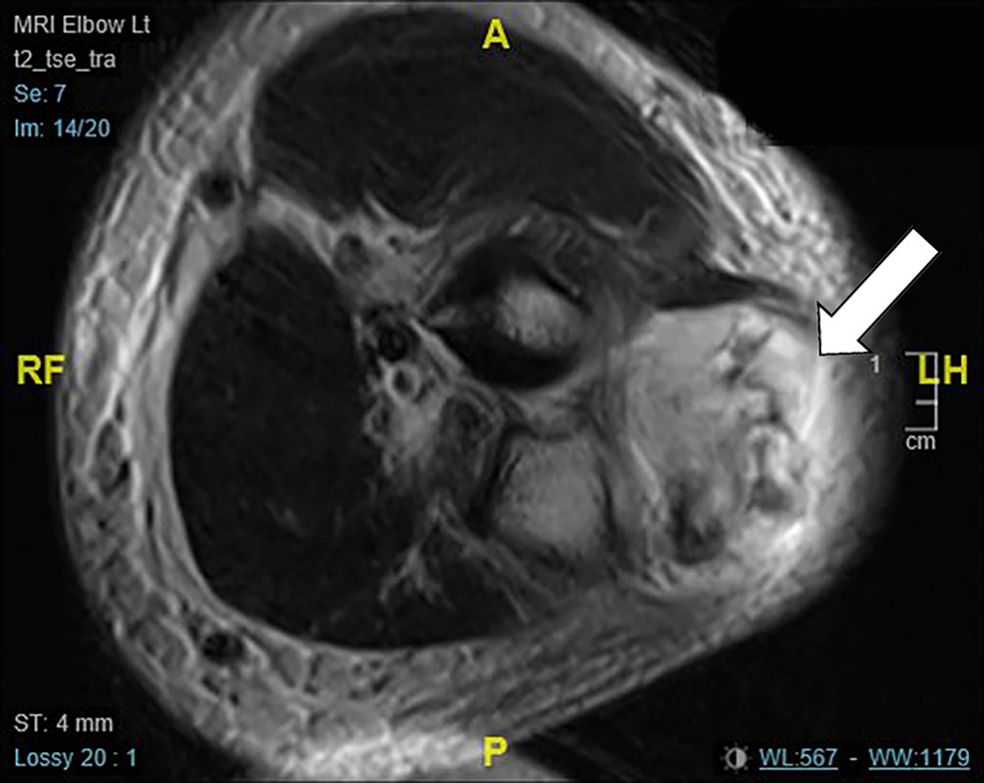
Initial MRI, white arrow points to the reported collection around 'foreign bodies'
